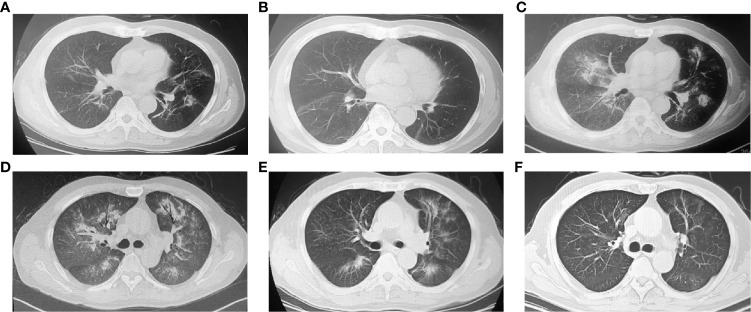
Chest computed tomography (CT) images showing the ICI-related pneumonitis (CIP) (A) ground-glass opacity in both lungs. (B) Inflammation absorbed after 2 weeks of glucocorticoid treatment. (C) CIP recurrence after resuming nivolumab treatment. Ground-glass opacity (GGO) reoccurred, and more lobes of the left lung were involved. (D) During the period of recurrent CIP, both lungs showed diffuse GGO, consolidation and the air bronchus-charging sign. (E) Image after 7 days of corticosteroid pulse therapy. The extent of CIP was reduced. (F) Image after 4 months of CIP treatment. The signs of pneumonitis subsided.
